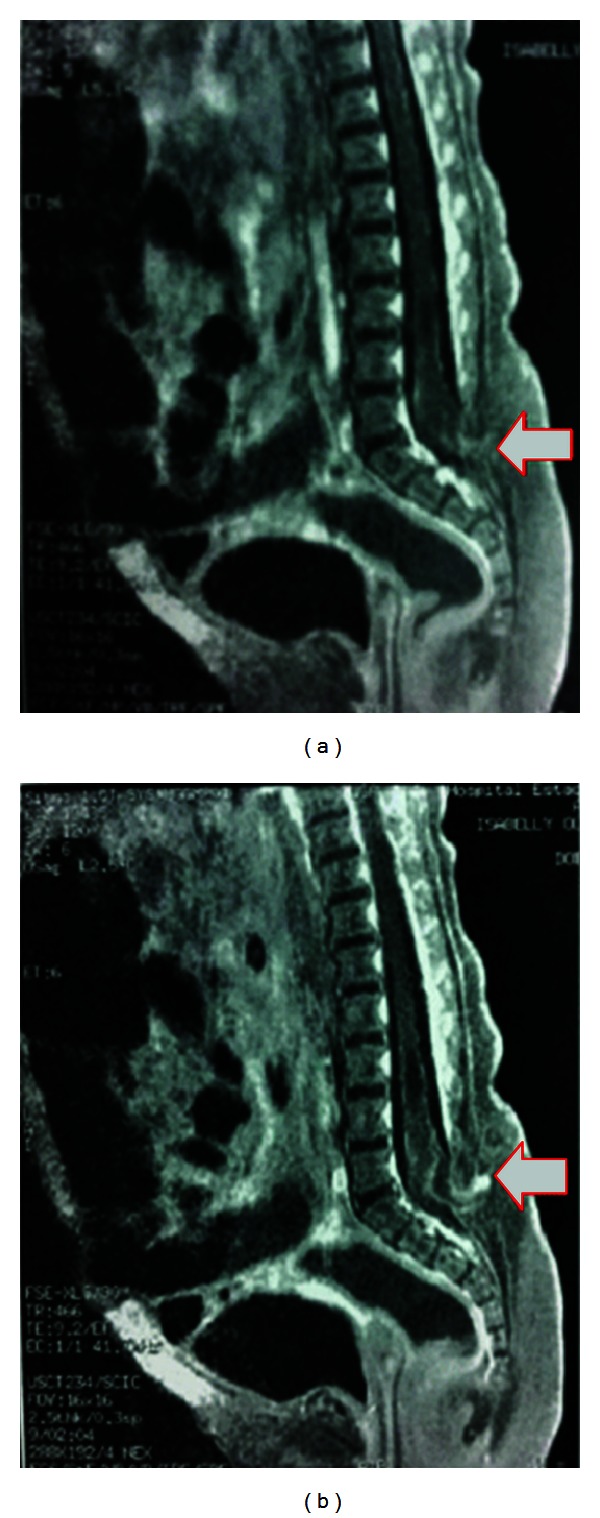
(a) and (b) Magnetic resonance imaging on the spine in the sagittal plane, showing a closure defect of the medullary canal at the level of the sacral region (S1) (white arrows), along with the presence of a posterior image with hypersignal in T1 and T2, compatible with a lipoma (red arrows).
